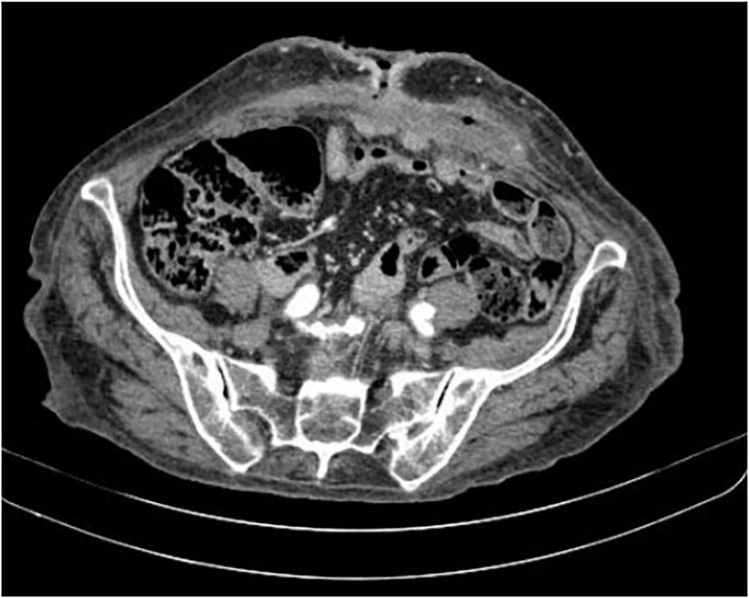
The axial CT section of the abdomen shows the loops in contact with the anterior abdominal wall at the level of the scar umbilical cord. The collection decreased compared with the previous CT scan in the subcutaneous tissue of the umbilical region.
